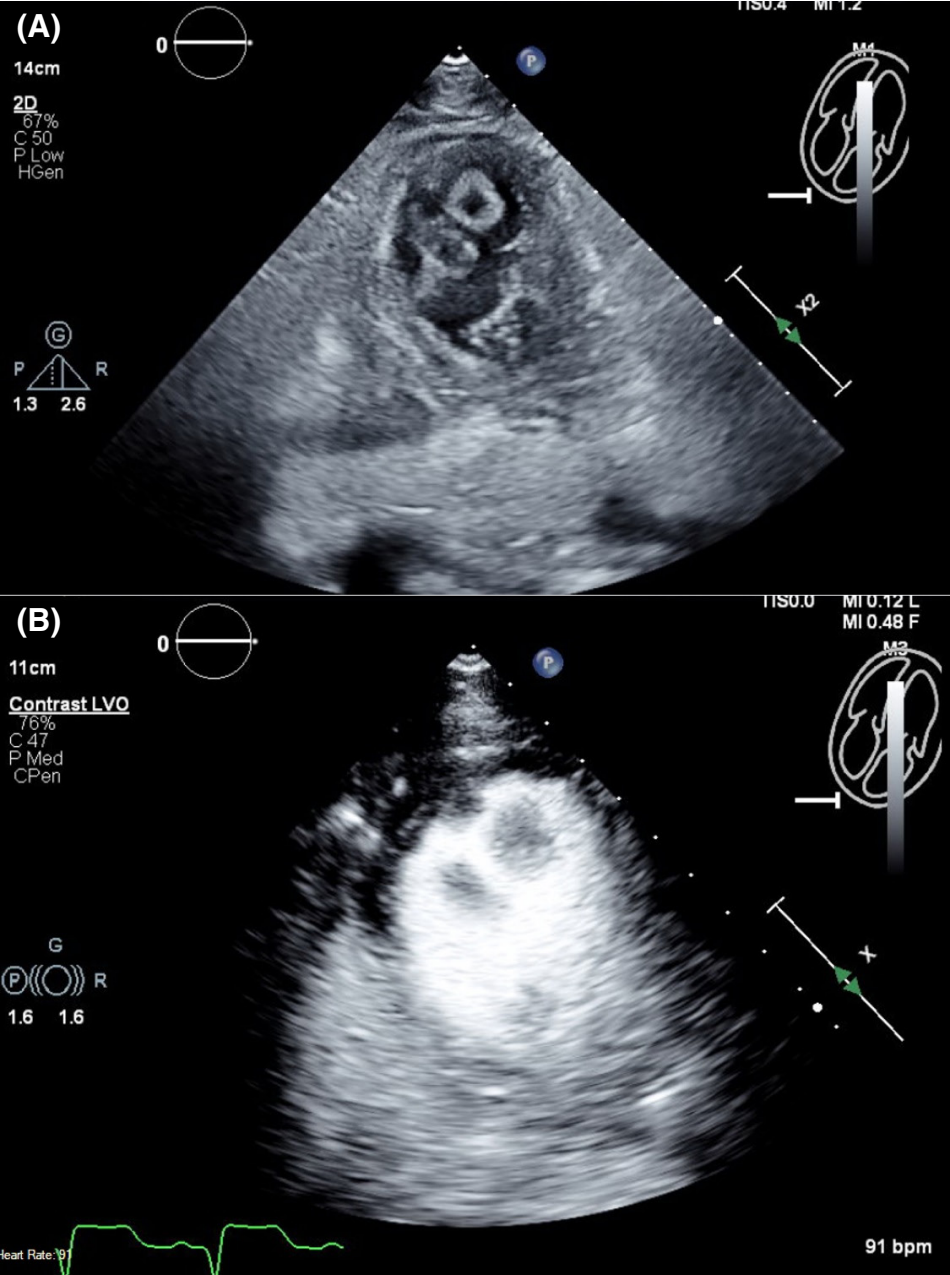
(A) TTE without contrast at the off‐axis apical 4 chamber view demonstrating multiple LV thrombi. (B) TTE with contrast at the off‐axis apical 4 chamber view demonstrating multiple LV thrombi. TTE, Transthoracic echocardiogram; LV, left ventricular
